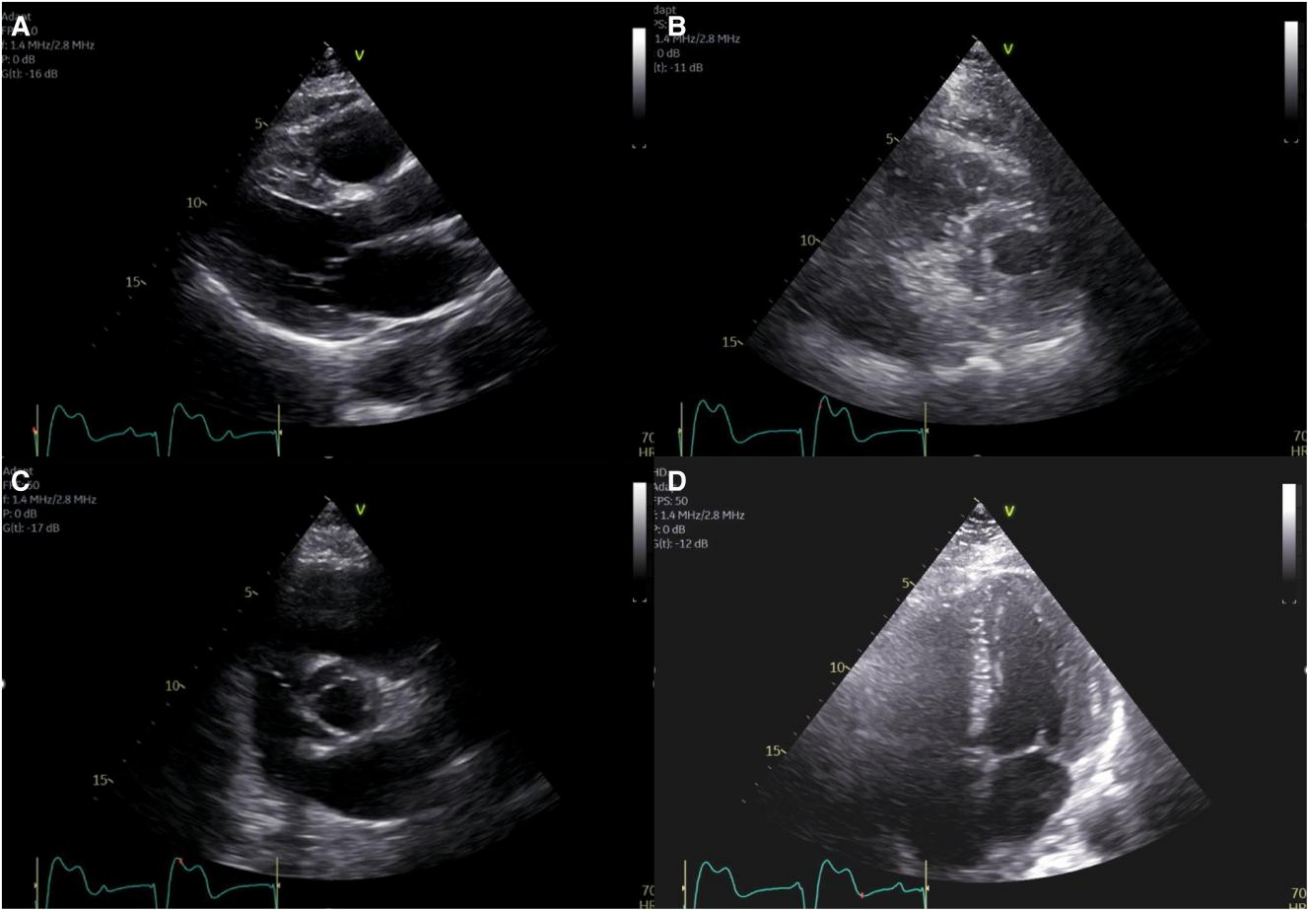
(*A*) Parasternal long axis view (PLAX) view: EF 55%, mildly enlarged left atrium (LA), and mild apical septal/inferior hypokinesis, likely due to temporary transvenous pacing. (*B*) parasternal short axis view (PSAX) view. (*C*) PSAX view of aortic valve: lead not visualized. (*D*) Apical four-chamber view.
